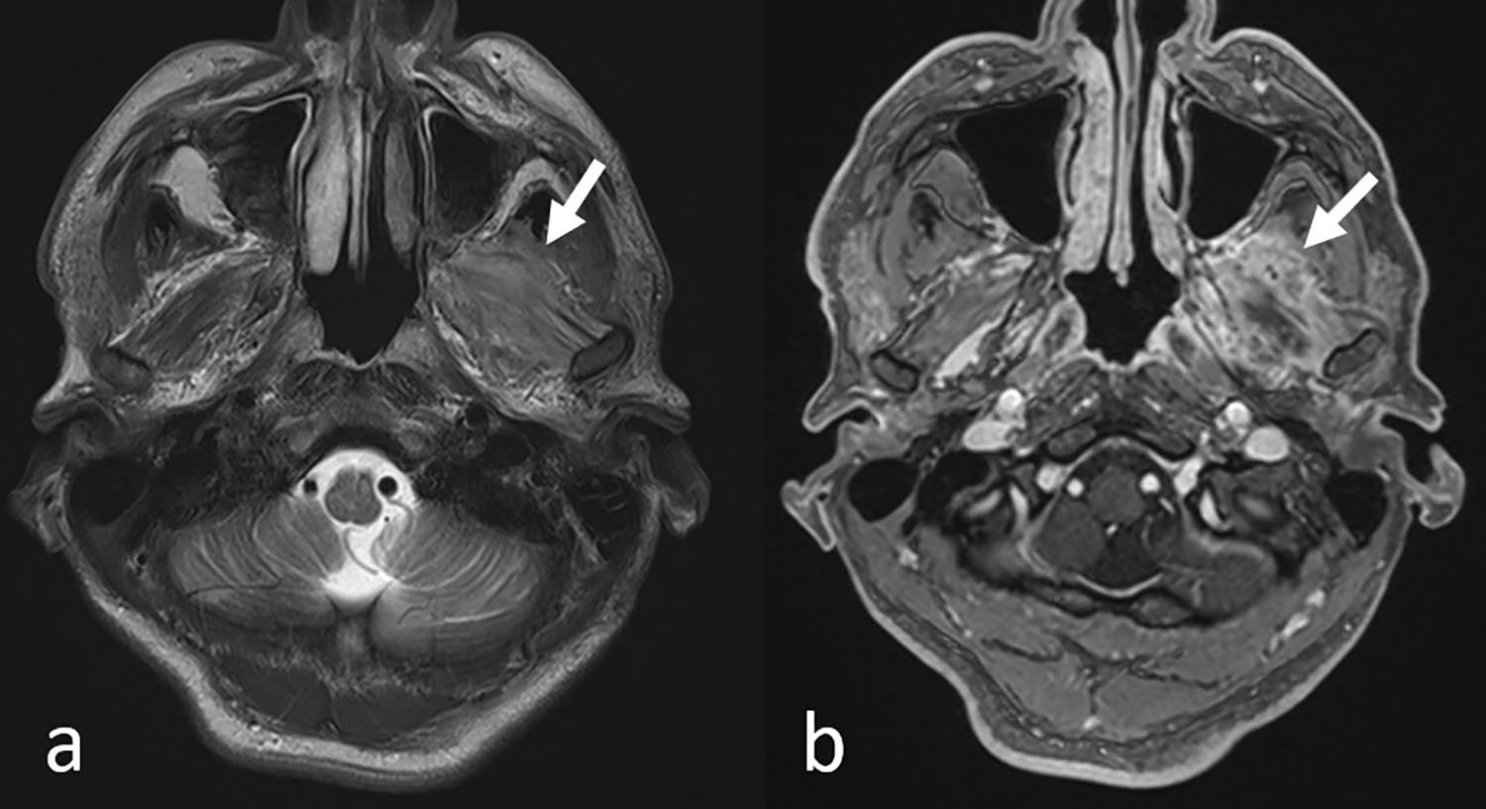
Diffuse left pterygoid muscle metastasis in a patient with esophageal cancer. T2-weighted image a shows that the swollen left pterygoid muscle has mildly higher intensity compared to the contralateral pterygoid muscle (arrow). Contrast-enhanced 3D fat-suppressed T1-weighted image b shows heterogeneous enhancement with an irregular margin of the swollen left pterygoid muscle (arrow)
